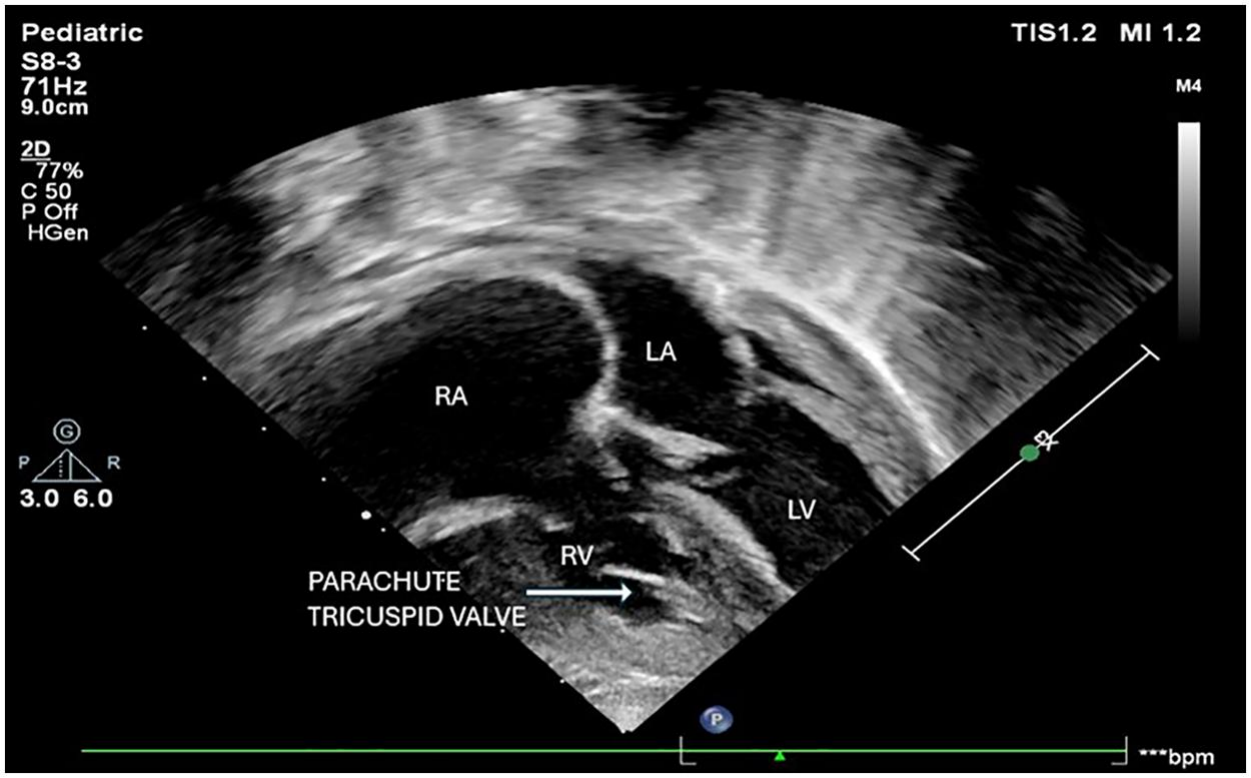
Apical four chamber view showing single papillary muscle on septal surface with chordal attachment from septal and anterior leaflets of tricuspid valve. Right atrium (RA), Right ventricle (RV), Left atrium (LA), Left ventricle (LV).
